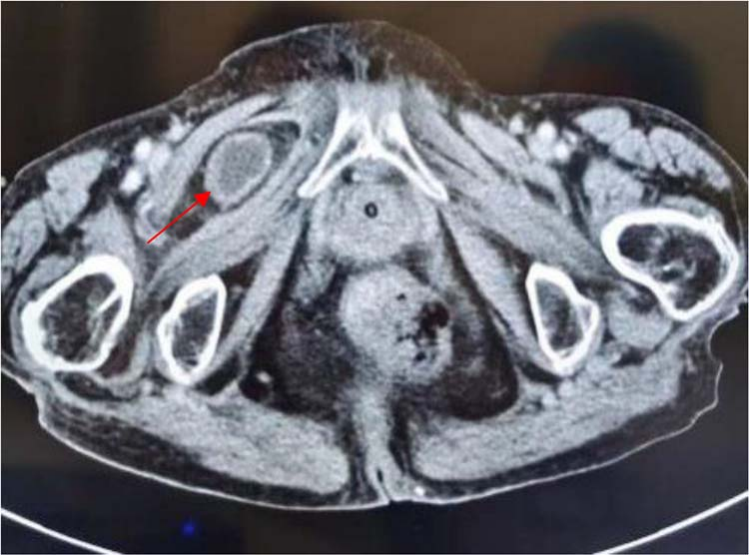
Computed tomography axis map showing the small intestine entering the femoral triangle (red arrow).
